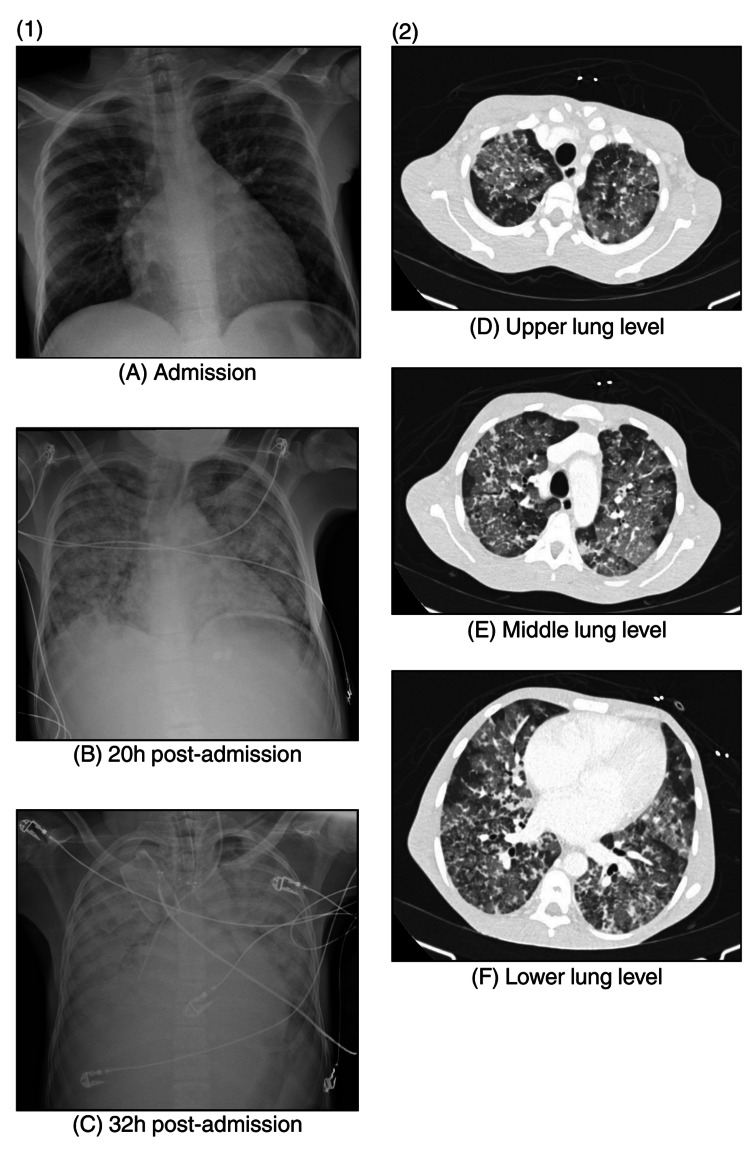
Progressive radiographic worsening of the chest showing new bilateral alveolar infiltrates (1) Sequential chest radiographs showing rapid progression from normal findings (A) to bilateral patchy infiltrates (B) and diffuse alveolar consolidation (C) within 30 hours, compatible with ACS. (2) High-resolution CT of the chest: axial images at the upper (D), middle (E), and lower (F) lung levels demonstrating diffuse bilateral ground-glass opacities with areas of confluent consolidation, a radiological pattern typical of ACS. ACS, acute chest syndrome
